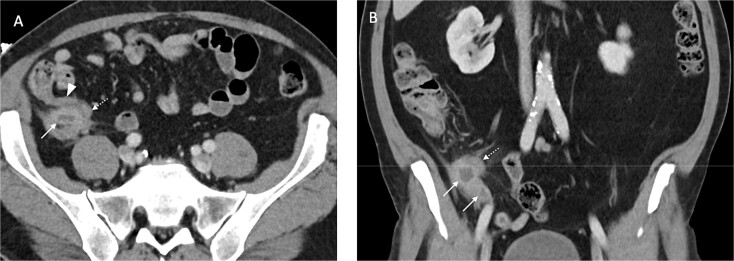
Contrast enhanced axial (A) and coronal (B) CT images in portal venous phase demonstrate periappendiceal abscesses (solid arrows) closely associated with thick-walled and inflamed tip of the appendix (dashed arrows). Note the normal appearing base of the appendix (arrowhead in A).
